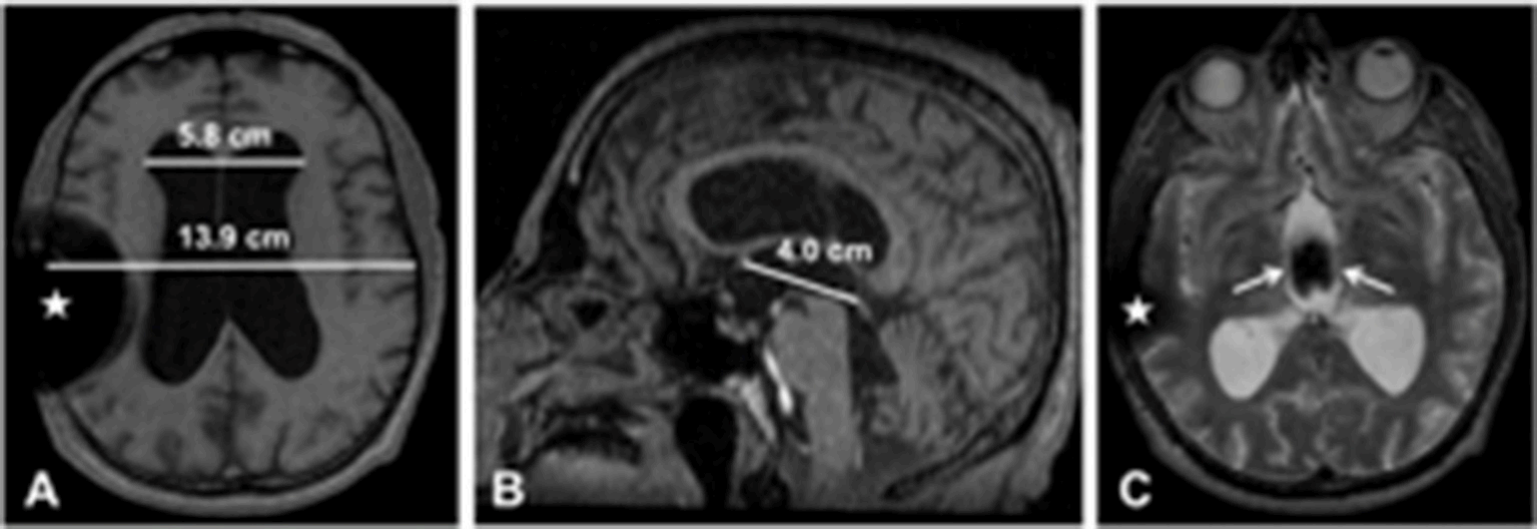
Brain MRI (A: T1 axial slice; B: T1 sagittal slice; C: T2 axial slice). (A) There is marked ventriculomegaly. The maximum width of the frontal horns of the lateral ventricles is 5.8 cm; the maximal internal diameter of the skull at the same level is 13.9 cm; the calculated Evans index is 0.42 (normal value: < 0.3). (B) The anterior commissure– posterior commissure line is drawn, and its length is 4.0 cm; this line is an important landmark for stereotactic targeting in GPi-DBS. (C) The third ventricle is also dilated, and there is prominent flow void artifact (white straight arrows); this artifact means that CSF flow velocity is high and there is no obstruction. Magnetic susceptibility artifacts due to ventriculoperitoneal catheter and valve (white stars).
